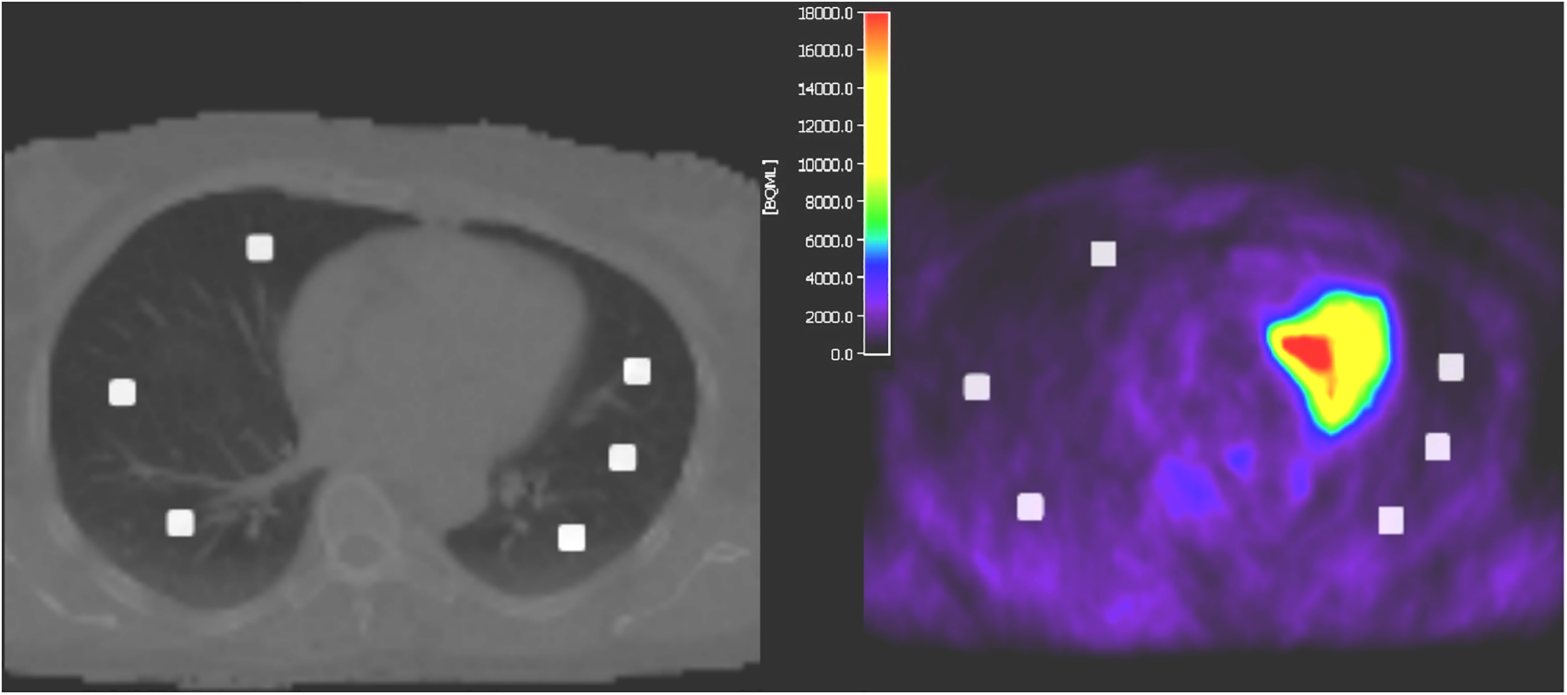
Example of ROI placements in the basal lung parts. Left hand side: ROIs superposed with coregistered μ-map from CT. Right hand side: ROIs superposed with the correlating PET.
